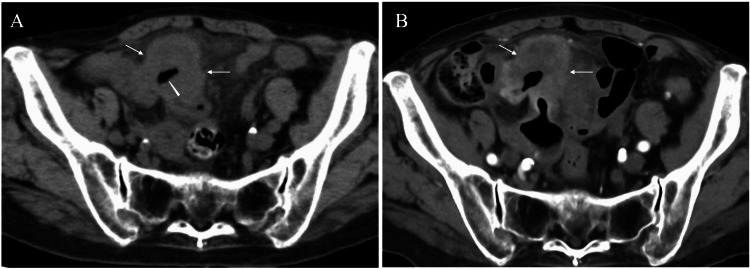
CT findings CT images showed an oval mass (arrows) with cavitation (arrowhead) in the lower abdomen (A) and a weak enhancement of the mass (arrows), especially in the peripheral areas of the mass (B) CT: computed tomography
